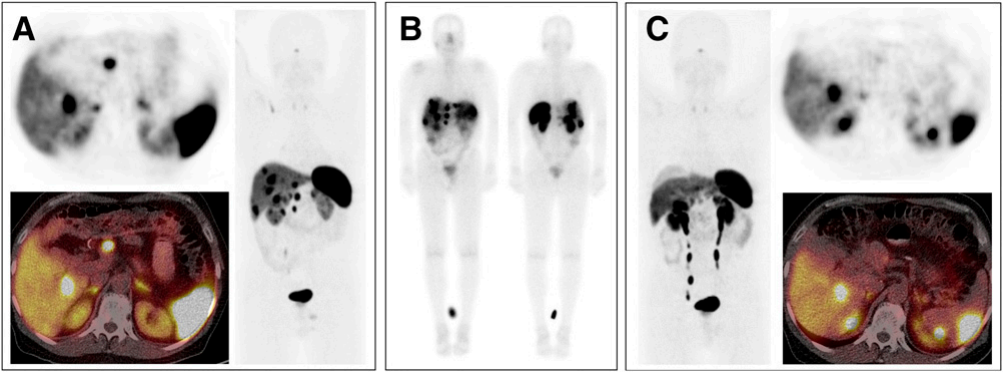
Representative examples of clinical PRRT images. PRRT in a 73-year-old patient with metastasized neuroendocrine cancer (G1). Pretherapeutic 68Ga-DOTANOC PET/computed tomography images show extensive metastases in the liver and additional abdominal lymph node metastases (A). Post-therapeutic whole-body scintigraphy after application of 177Lu-DOTATATE confirms uptake in metastatic lesions (B). After four cycles of 177Lu-DOTATATE, 68Ga-DOTANOC PET/computed tomography demonstrates considerable response of liver and abdominal lymph node metastases (C). Images courtesy of M. Eiber, Department of Nuclear Medicine, Technical University Munich, Germany.
